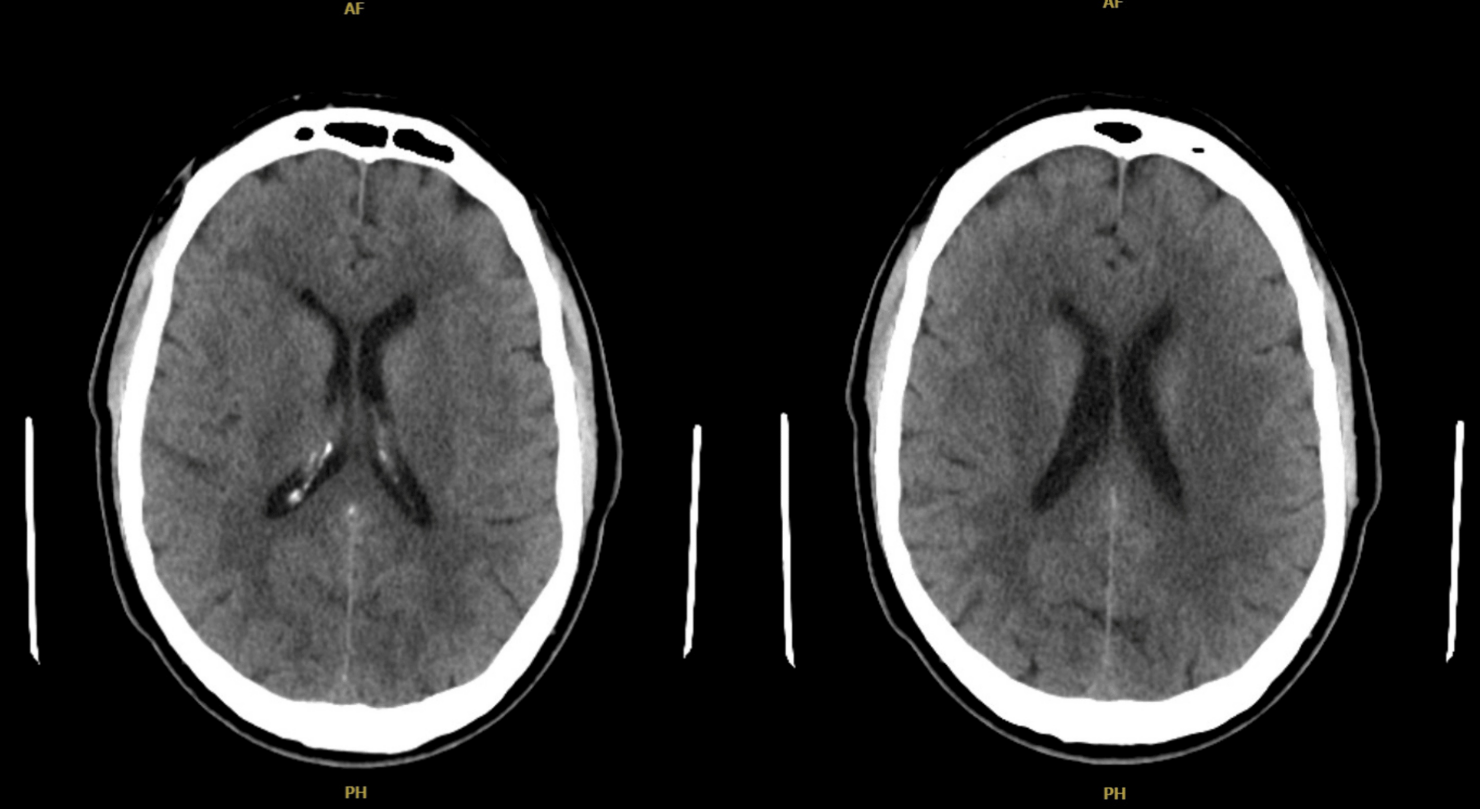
Axial CT of the brain showing normal brain parenchyma and ventricles. CT: computed tomography
